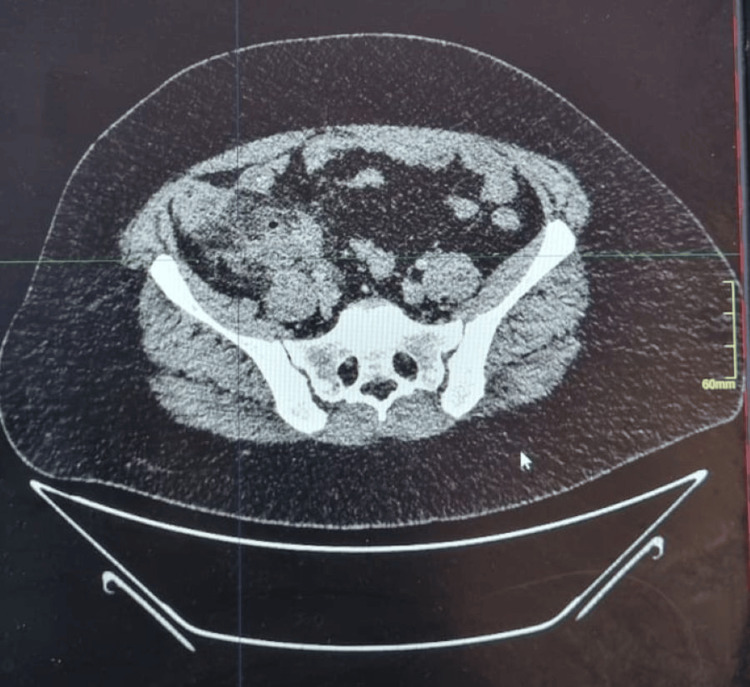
Preoperative contrast-enhanced CT of the abdomen and pelvis demonstrating significant inflammatory changes in the right iliac fossa CT: computed tomography
